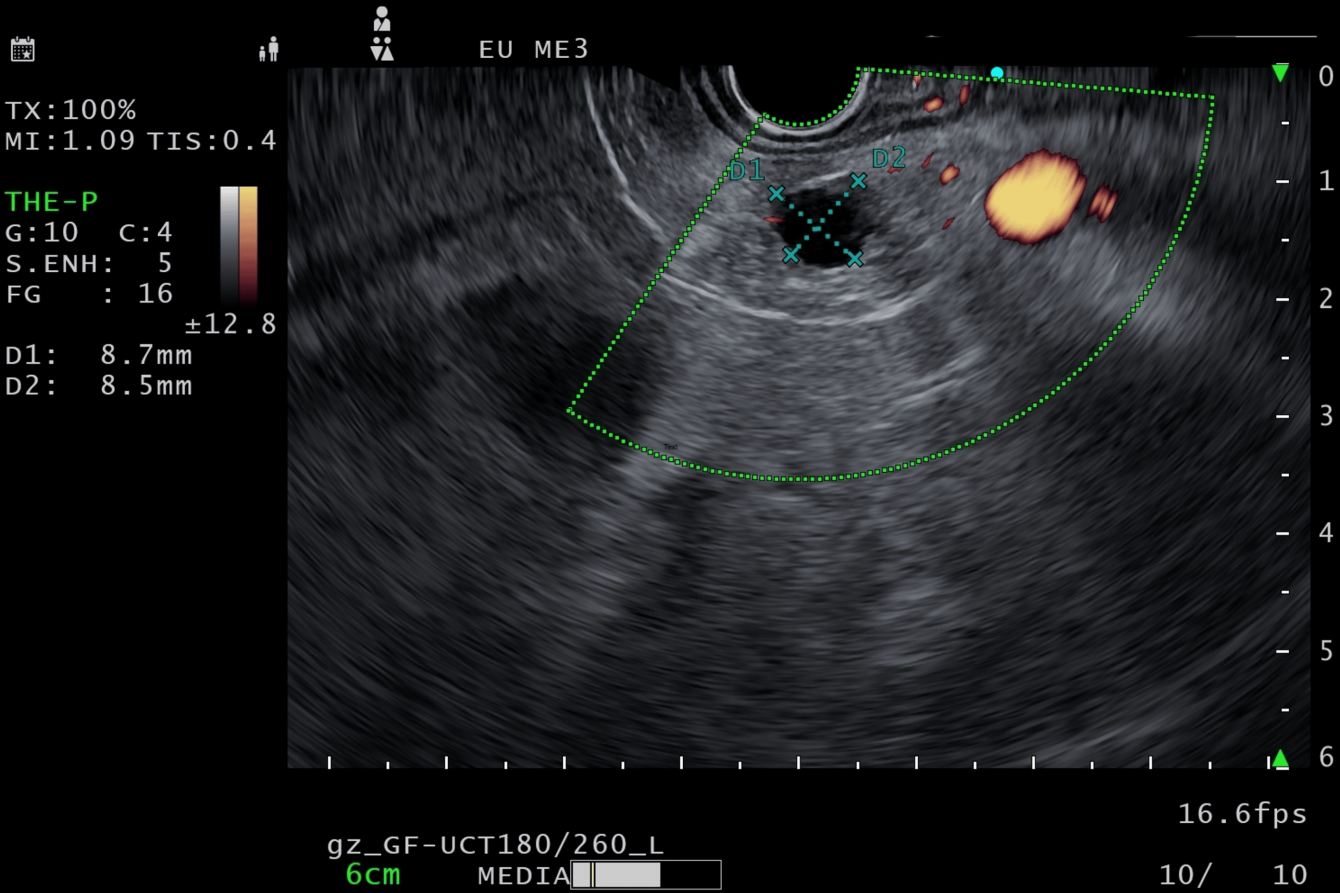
EUS localization of VIPoma EUS: Endoscopic ultrasound
